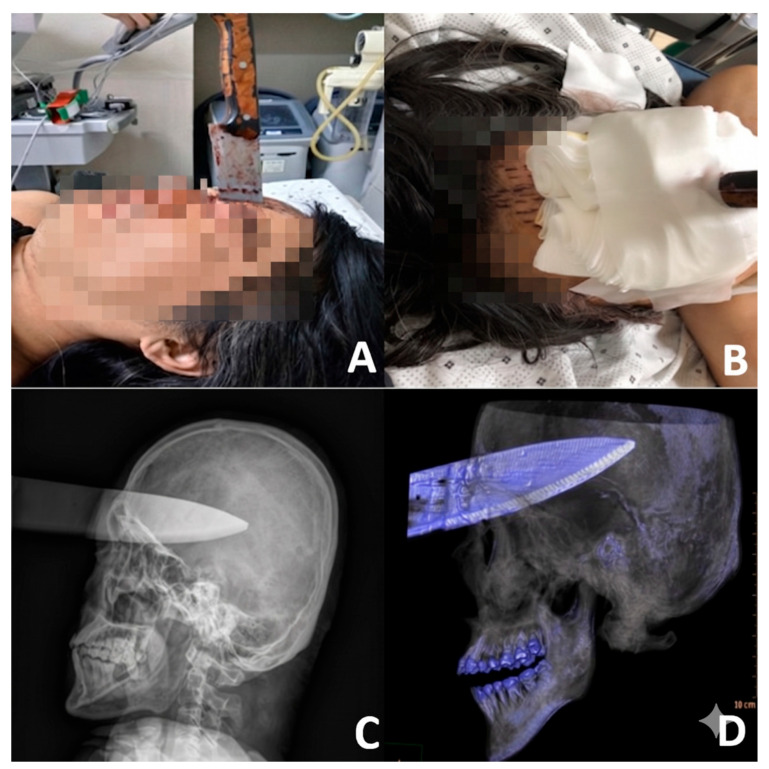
Preoperative clinical and radiographic findings. (A) Clinical photograph showing the vertical penetration of a kitchen knife in the midline glabellar region. (B) Superior view of the frontal scalp revealing multiple superficial “hesitation marks,” indicating failed attempts to penetrate the calvarium before the successful trans-sinus entry. (C) Lateral skull radiograph clearly demonstrating the angle and depth of the blade traversing the frontal sinus. (D) Sagittal view of the brain computed tomography (CT) scan (bone window) revealing the trajectory of the knife passing through the frontal sinus and entering the anterior cranial fossa.
